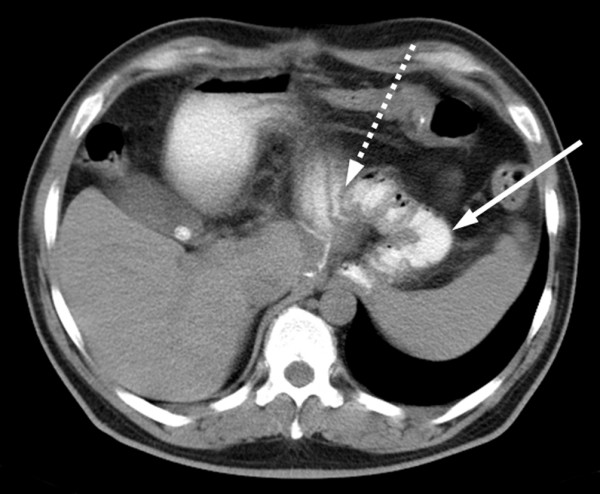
Postoperative CT-Scan. Postoperative follow-up CT scan after 18 months showing the jejunal interposition (gastro-jejunostomy: interrupted arrow).
